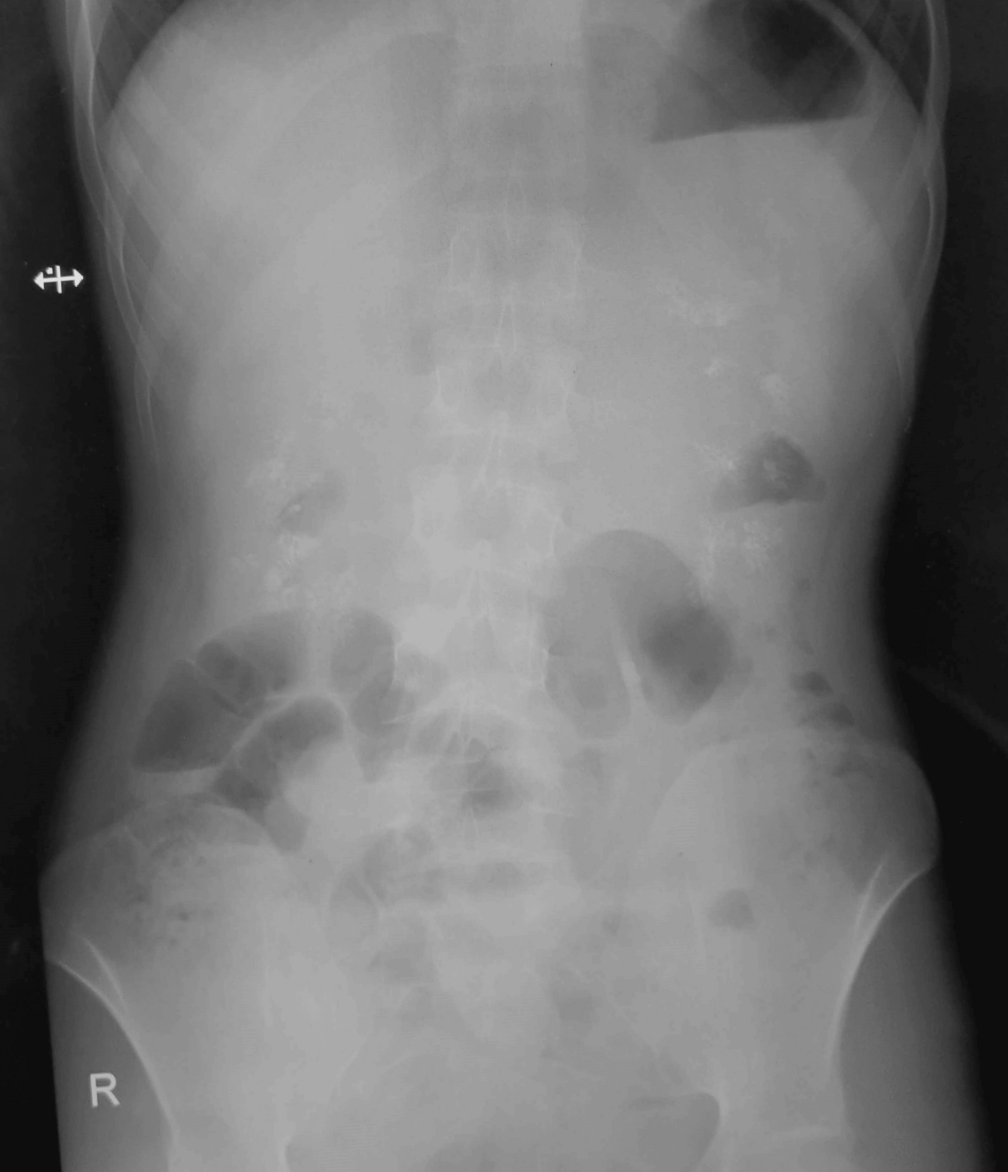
X-ray abdomen anteroposterior (AP) view of the patient showing diffuse increase in radiodensity observed in the bilateral kidneys consistent with nephrocalcinosis with no evidence of calculi in the ureters or urinary bladder or radiographic signs of urinary tract obstruction
